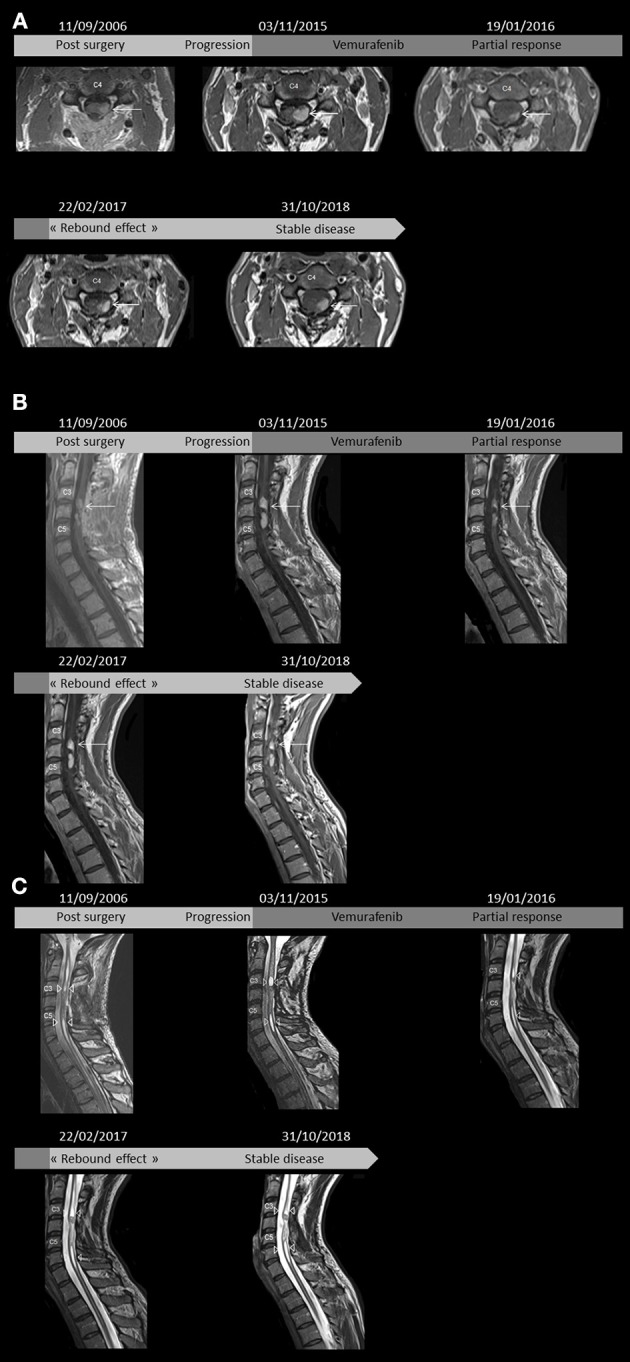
Axial T1-weighted images between C3 and C4 of T1-weighted cervical spine magnetic resonance imaging (MRI) after gadolinium injection administration show dominant, patchy intense enhancing left-sided lesion within the spinal cord (arrows) 1 month after surgery, in pre-treatment with vemurafenib, 8 weeks after the beginning of vemurafenib, 2 months after vemurafenib discontinuation, and last follow-up (A). Sagittal post contrast T1-weighted images revealed lesions between C3 and C4 as well as C4 and C5 (arrows) 1 month after surgery, in pre-treatment, 8 weeks after beginning treatment, 2 months after discontinuation, and last follow-up (B). Sagittal T2-weighted images showing syringomyelia (arrowheads) rostral and caudal to the intramedullary tumor 1 month after surgery, at pre-treatment, 8 weeks after beginning treatment, 2 months after discontinuation, and last follow-up. Note the T2 hyperintensity in the spinal cord above and below the syringomyelia without associated enhancement (C).
